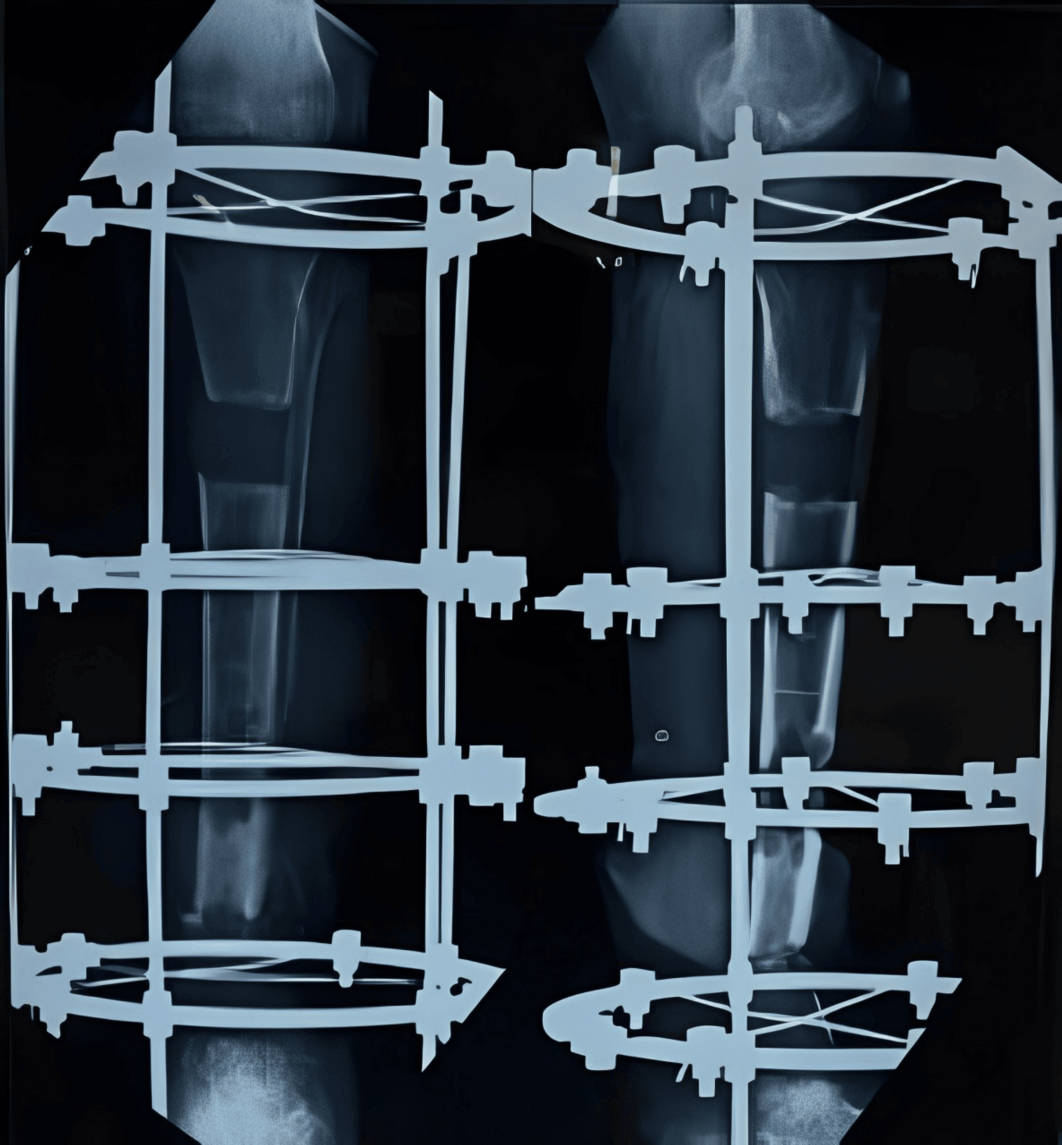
X-ray after eight weeks of distraction
